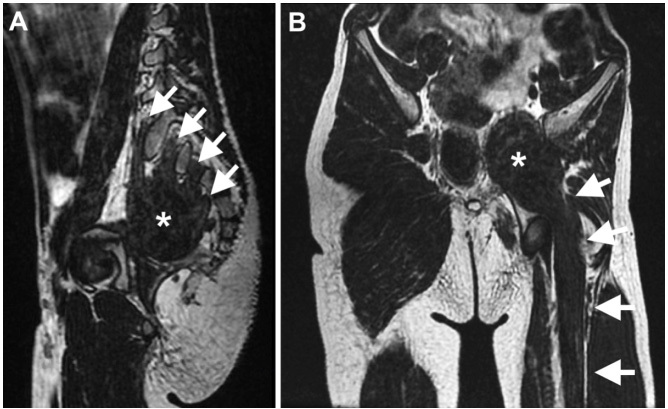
Magnetic resonance imaging studies of the pelvis. Oblique sagittal reformatted T2-weighted MR image of the pelvis shows contribution of the left L5 to S3 nerve roots (white arrows) to form the pelvic mass (asterisk) (A). Oblique coronal reformatted T2-weighted MR image of the pelvis shows continuity of the pelvic mass (asterisk) with the enlarged sciatic nerve (arrows) and the similarity of its signal intensity with the adjacent muscle bellies (B).
